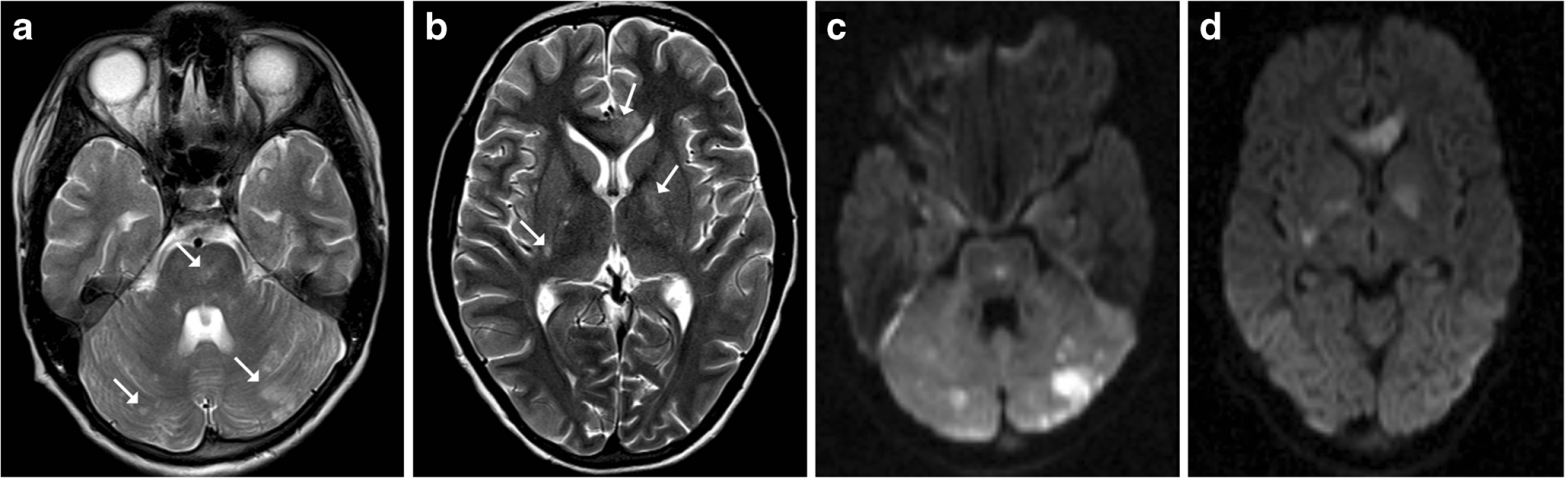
A 13-year-old patient with cerebral malaria: Axial T2-weighted MRI reveals hyperintense foci showing diffusion restriction in the left cerebellum, pons, bilateral basal ganglia and corpus callosum (*arrows*) corresponding to areas of cytotoxic oedema
